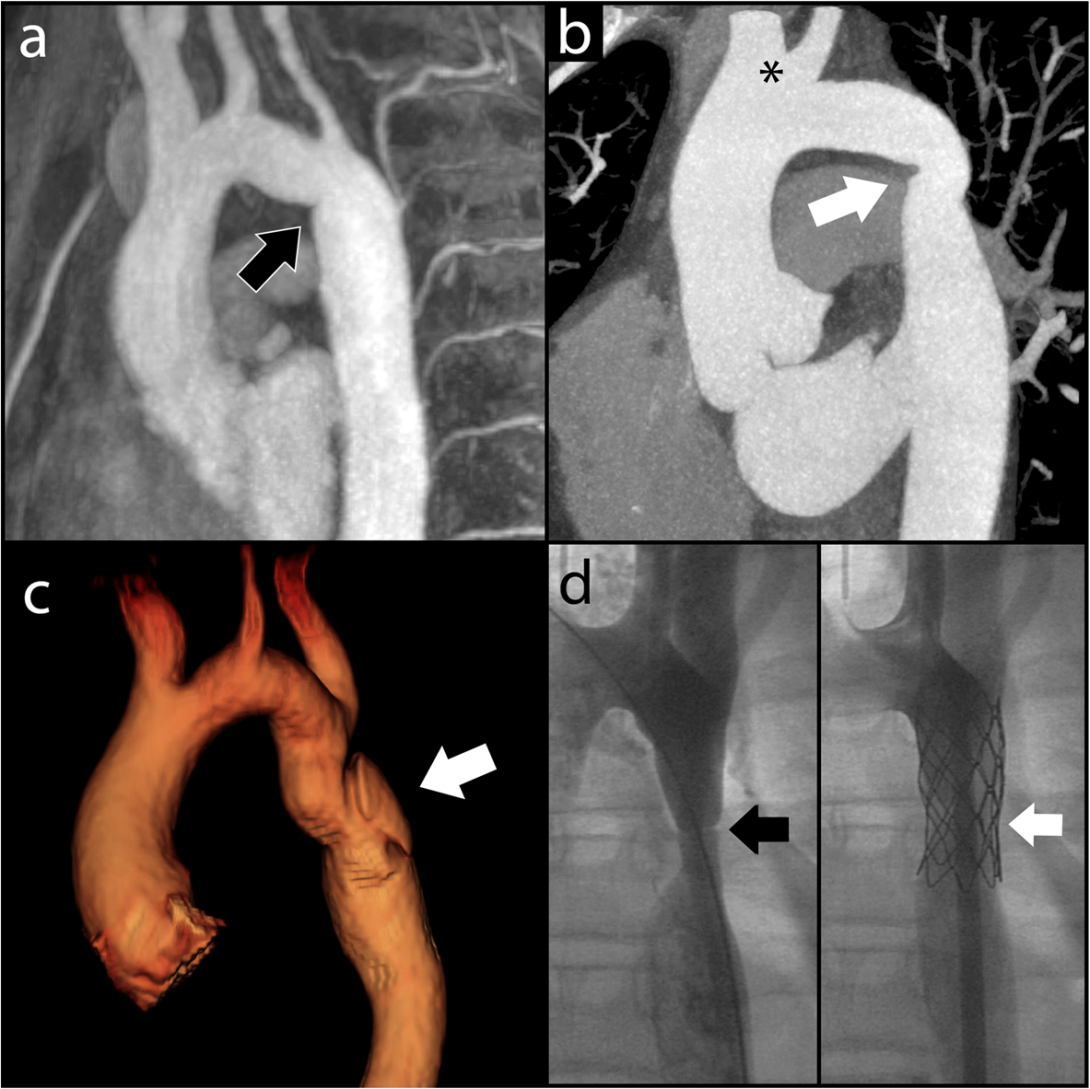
Examples of CoA repair. a Oblique sagittal maximum intensity projection reconstruction of MRA performed for a patient who underwent end-to-end anastomotic CoA repair. A mild fold is demonstrated at the CoA repair site at the aortic isthmus (arrow). b Oblique sagittal maximum intensity projection reconstruction CT angiogram for patient with subclavian flap CoA repair. There is absence of the proximal left subclavian artery with mild narrowing at the site of coarctation repair in the distal arch (arrow). Note normal variant conjoint origin of the right brachiocephalic and left common carotid artery (asterisk). c 3D reconstruction MRA in a patient who underwent patch repair of significant CoA. The white arrow indicates a pseudoaneurysm in the proximal descending aorta, which developed at the site of repair. d Fluoroscopic images of CoA stent procedure. Left panel shows CoA in the proximal descending aorta (black arrow). Right panel shows successful stent implantation with improved patency of the proximal descending aorta (white arrow)
